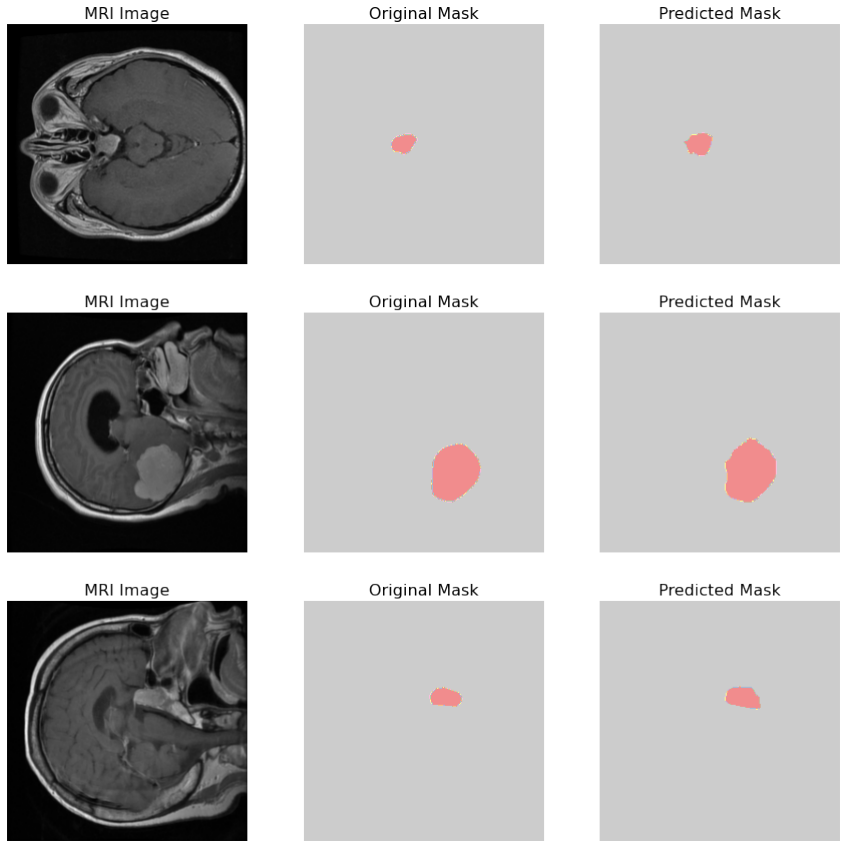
Visual comparison showcasing the original MRI image alongside its corresponding true mask and the predicted mask generated by the proposed model.
